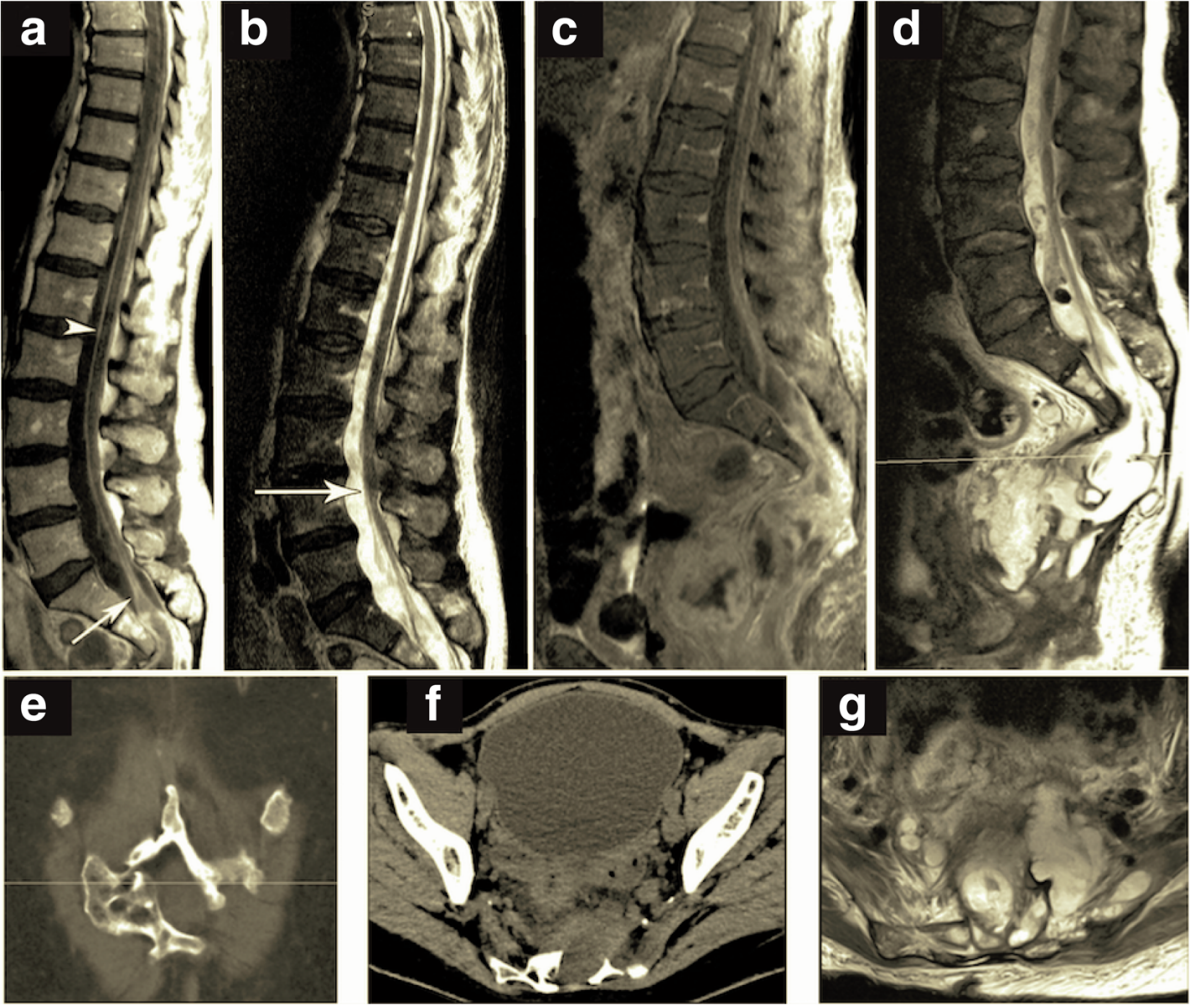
Neuroradiological Imaging. MRI imaging (a sagittal T1-SE post-contrast; b sagittal T2-TSE: sagittal fat-saturated T1-SE post-contrast; d sagittal T2-TSE, e coronally reconstructed CT; f axially reconstructed CT at the level marked by a line in d; g axial T2-TSE sequence at the level marked by a line in c). (a, c) Strong contrast enhancement of the meningeal structures due to the meningitis are shown in the post-contrast MRI series. The arrowhead in A exemplarily points at the strongly enhancing surface of the spinal cord. The arrow in A points at the contrast-enhancing filum terminale. (b) The level of the medullary cone is at the level of the vertebrates L3 and L4, which is unusually low, see arrow. (c) The distended rectum and a connection to the spinal canal are visible. The meningocele cannot be unequivocally delineated from the rectal carcinoma. (d, e) CT at the level marked by a line in d; (f) axial sequence at the level marked by a line in c) demonstrating a sacral menigocele with tethering of the spinal cord and bony dysraphism
